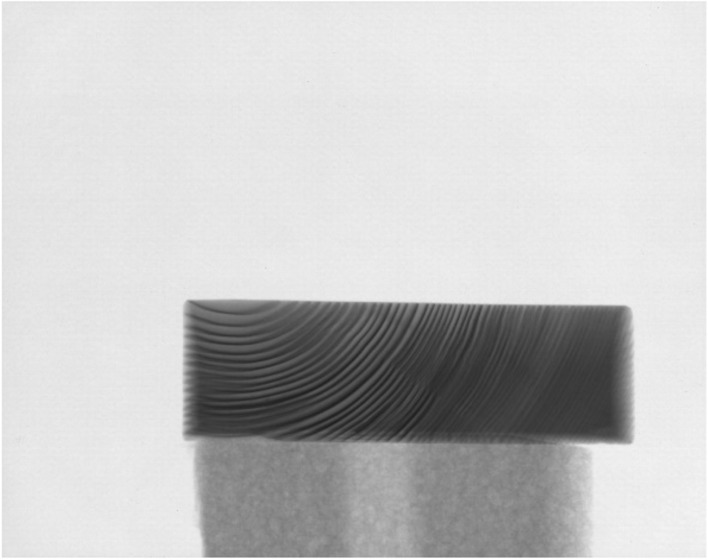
A radiograph of a wooden block, with the transverse section facing the source.
